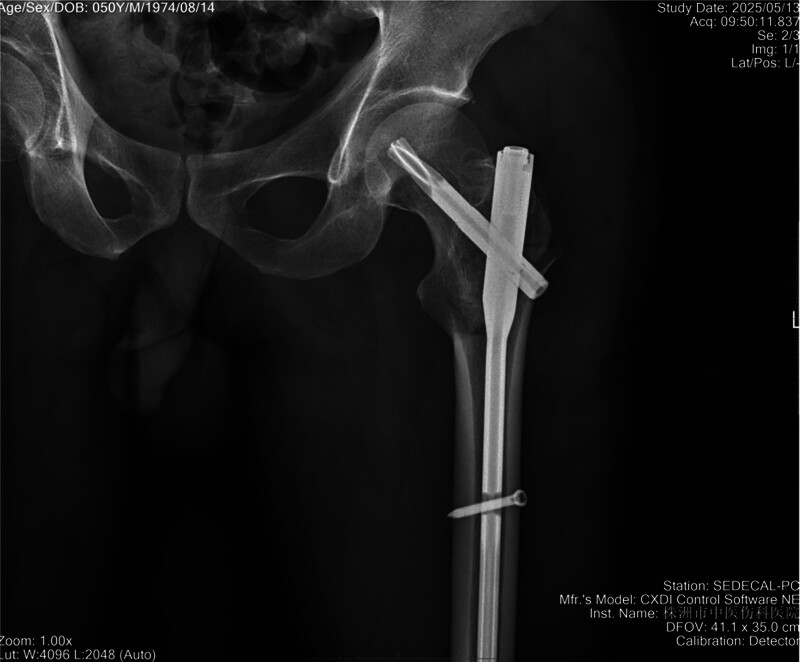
An anteroposterior radiograph of left hip was reviewed postoperatively.
